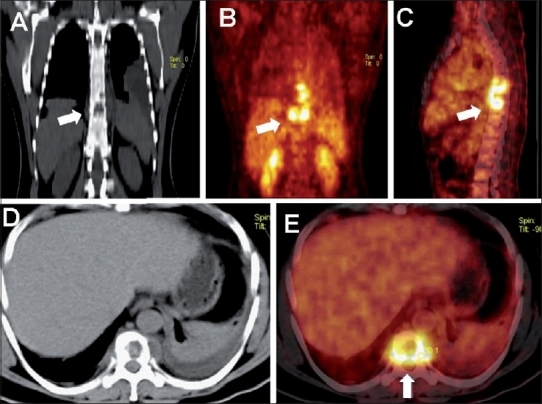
Tuberculosis of the spine. A coronal plain CT reconstruction (A) in a 42-year-old man with upper back pain shows osteolytic lesions involving multiple contiguous mid-dorsal vertebrae (arrows), with a paravertebral cold abscess and a left-sided pleural effusion, suggesting tuberculosis. Coronal (B) and sagittal (C) PET with axial plain CT (D), and corresponding PET/CT (E) images acquired to evaluate the extent of the disease show intense FDG uptake in the affected thoracic vertebrae. No other FDG-avid lesion is detected. The patient responded well to anti-tuberculous therapy
